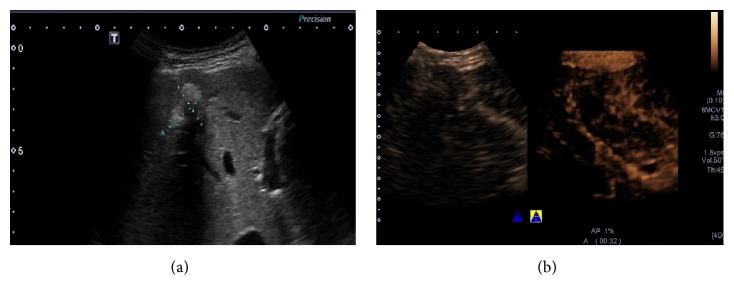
Presence of attenuation due to RFA treatment. B mode ultrasound image in a 71-year-old man showed a 25 mm sized lesion treated by RFA in the right lobe of the liver. It had posterior attenuation caused by scars or necrosis (a). Its posterior border cannot be demonstrated by 2D-CEUS (left), whereas it can be shown on dynamic 3D-CEUS. The relationship of the lesion and the posterior adjacent large vessels can also be shown (b).
